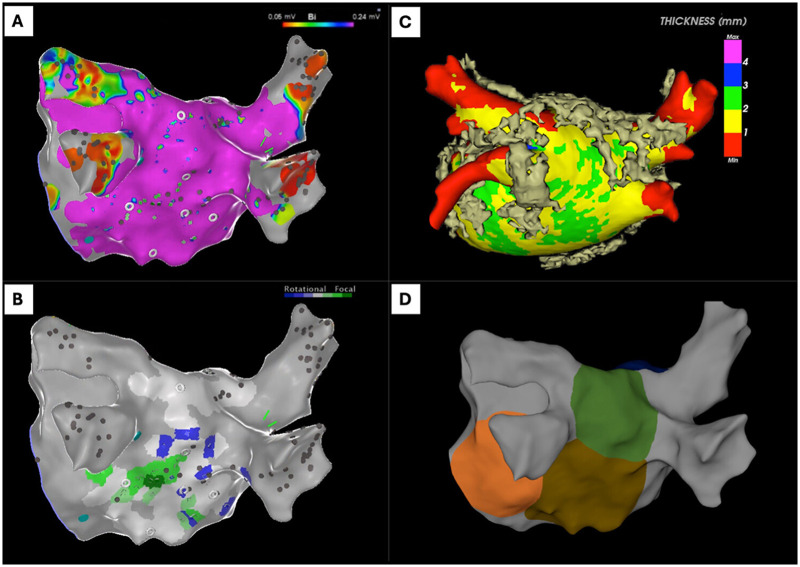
Multidimensional assessment of left atrium. (A) Bipolar voltage mapping with CARTO (B) CARTOFINDER map (C) Pre-procedural multidetector computed tomography processed with ADAS3D showing wall thickness and epicardial adipose tissue (D) Segmentation performed with CARTONET.
